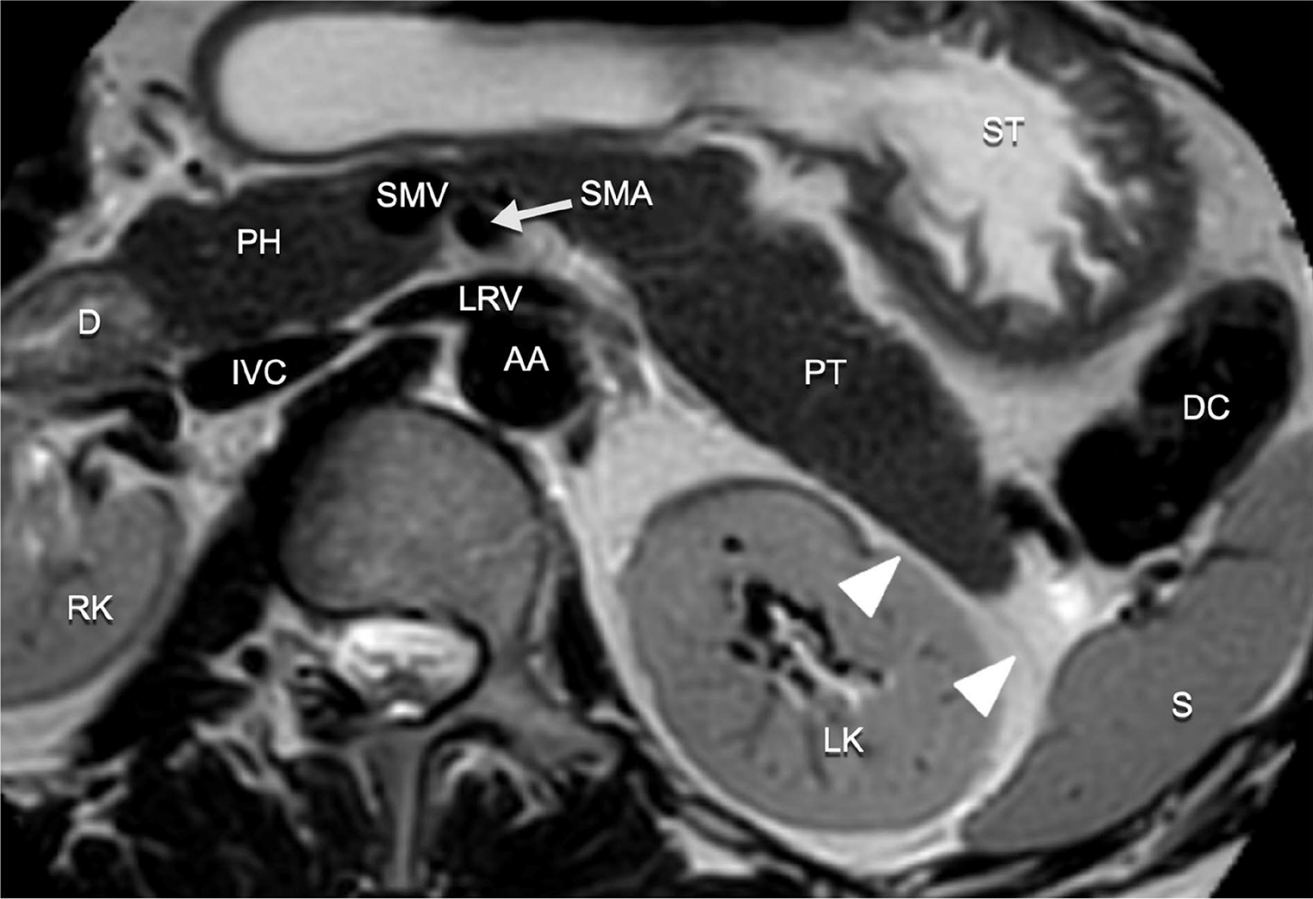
T2-weighted MR image of the pancreatic tail. The anterior renal fascia (arrow heads) is in close proximity to the pancreatic tail. AA: abdominal aorta, D: duodenum (descending part), DC: descending colon, IVC: inferior vena cava, LK: left kidney, LRV: left renal vein, PH: pancreatic head, PT: pancreatic tail, RK: right kidney, S: spleen, SMA: superior mesenteric artery, SMV: superior mesenteric vein, ST: stomach
